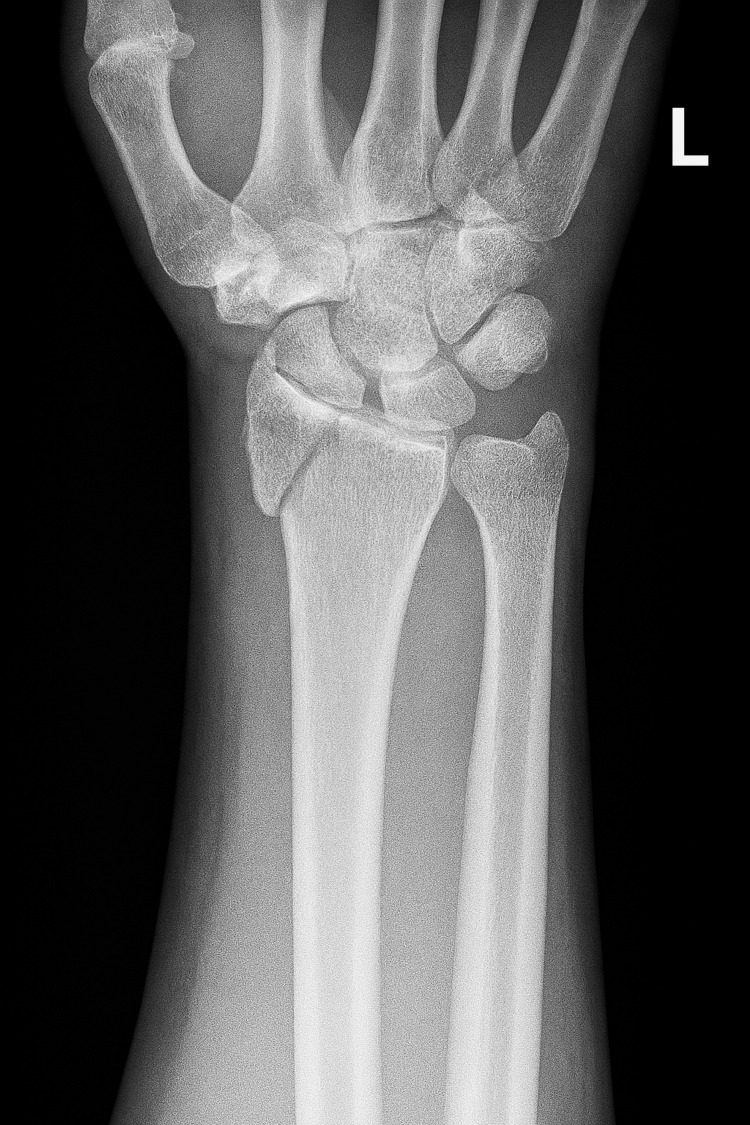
Radiograph showing a mildy displaced acute-to-subacute left intra-articular fracture at the distal radiocarpal joint
